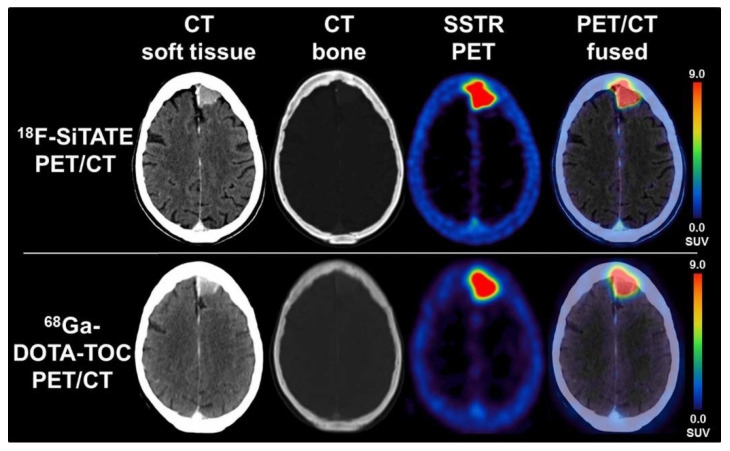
PET/CT comparison between [^18^F]SiTATE and [^68^Ga]Ga-DOTA-TOC in a patient with a falx meningioma with transosseous extension. Images show preferable resolution with [^18^F]SiTATE, therefore yielding a higher quality image. Reprinted with permission from Ref. [34]. Copyright 2021 Wolters Kluwer Health, Inc.
